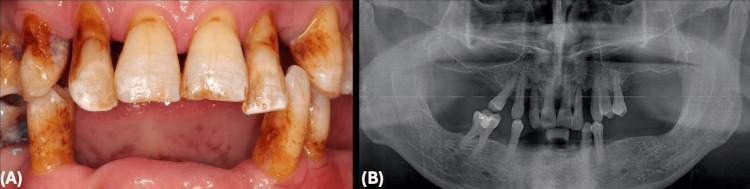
Initial Presentation (A) Clinical presentation at the initial visit. (B) Panoramic radiographic presentation at the initial visit
